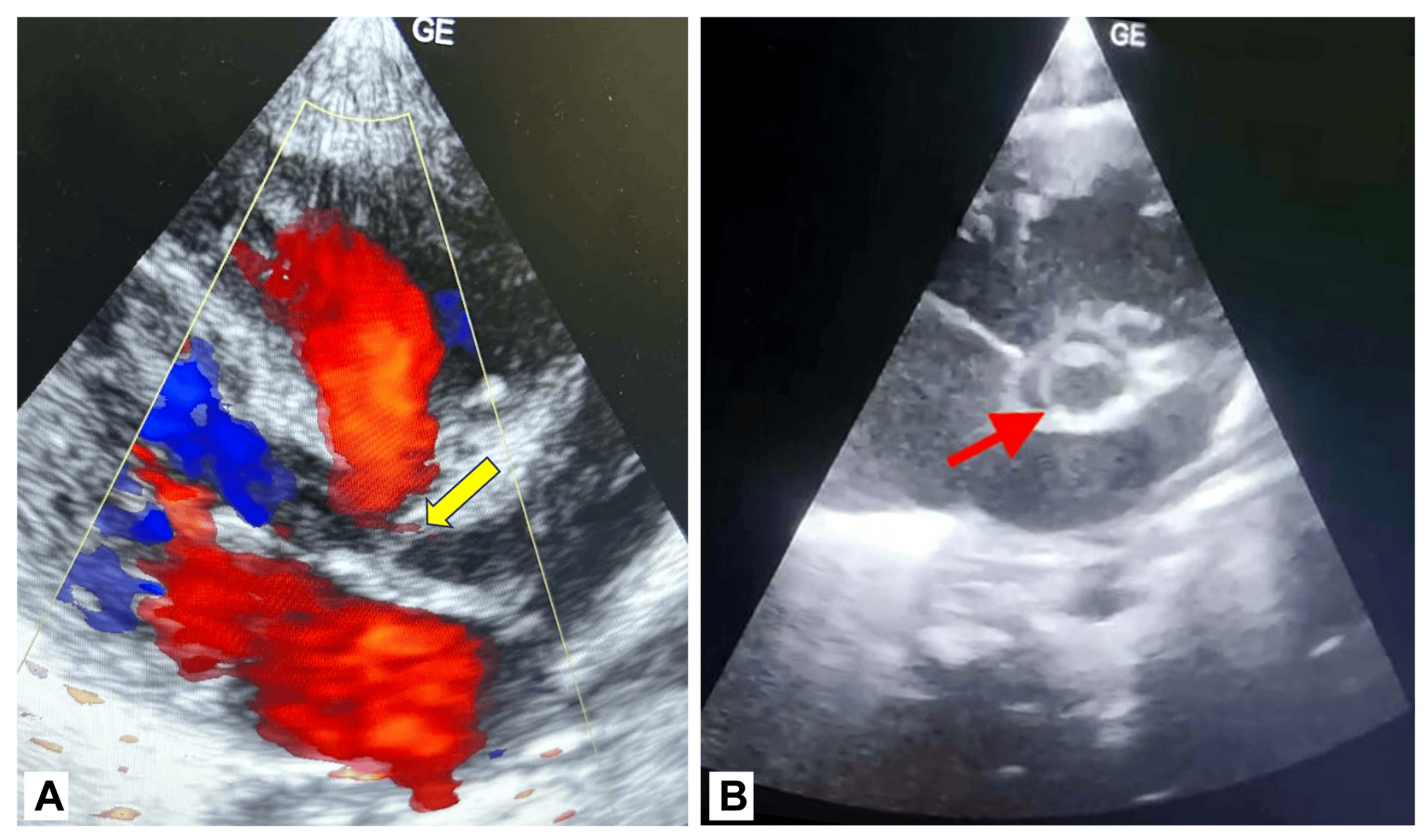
A) Two-dimensional echocardiography (parasternal long axis view) showing aortic stenosis (yellow arrow); B) Two-dimensional echocardiography (short axis view) showing bicuspid aortic valve (red arrow).
